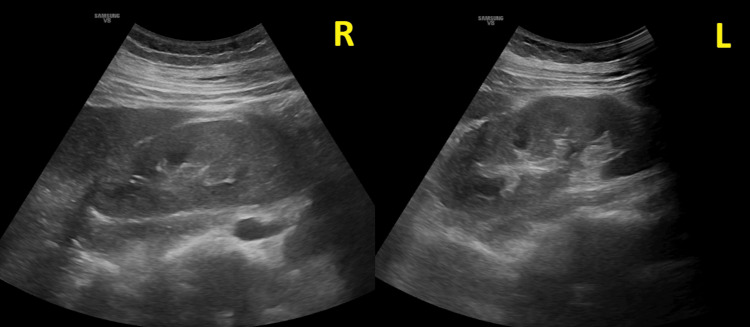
Ultrasound of right (R) and left (L) kidneys
